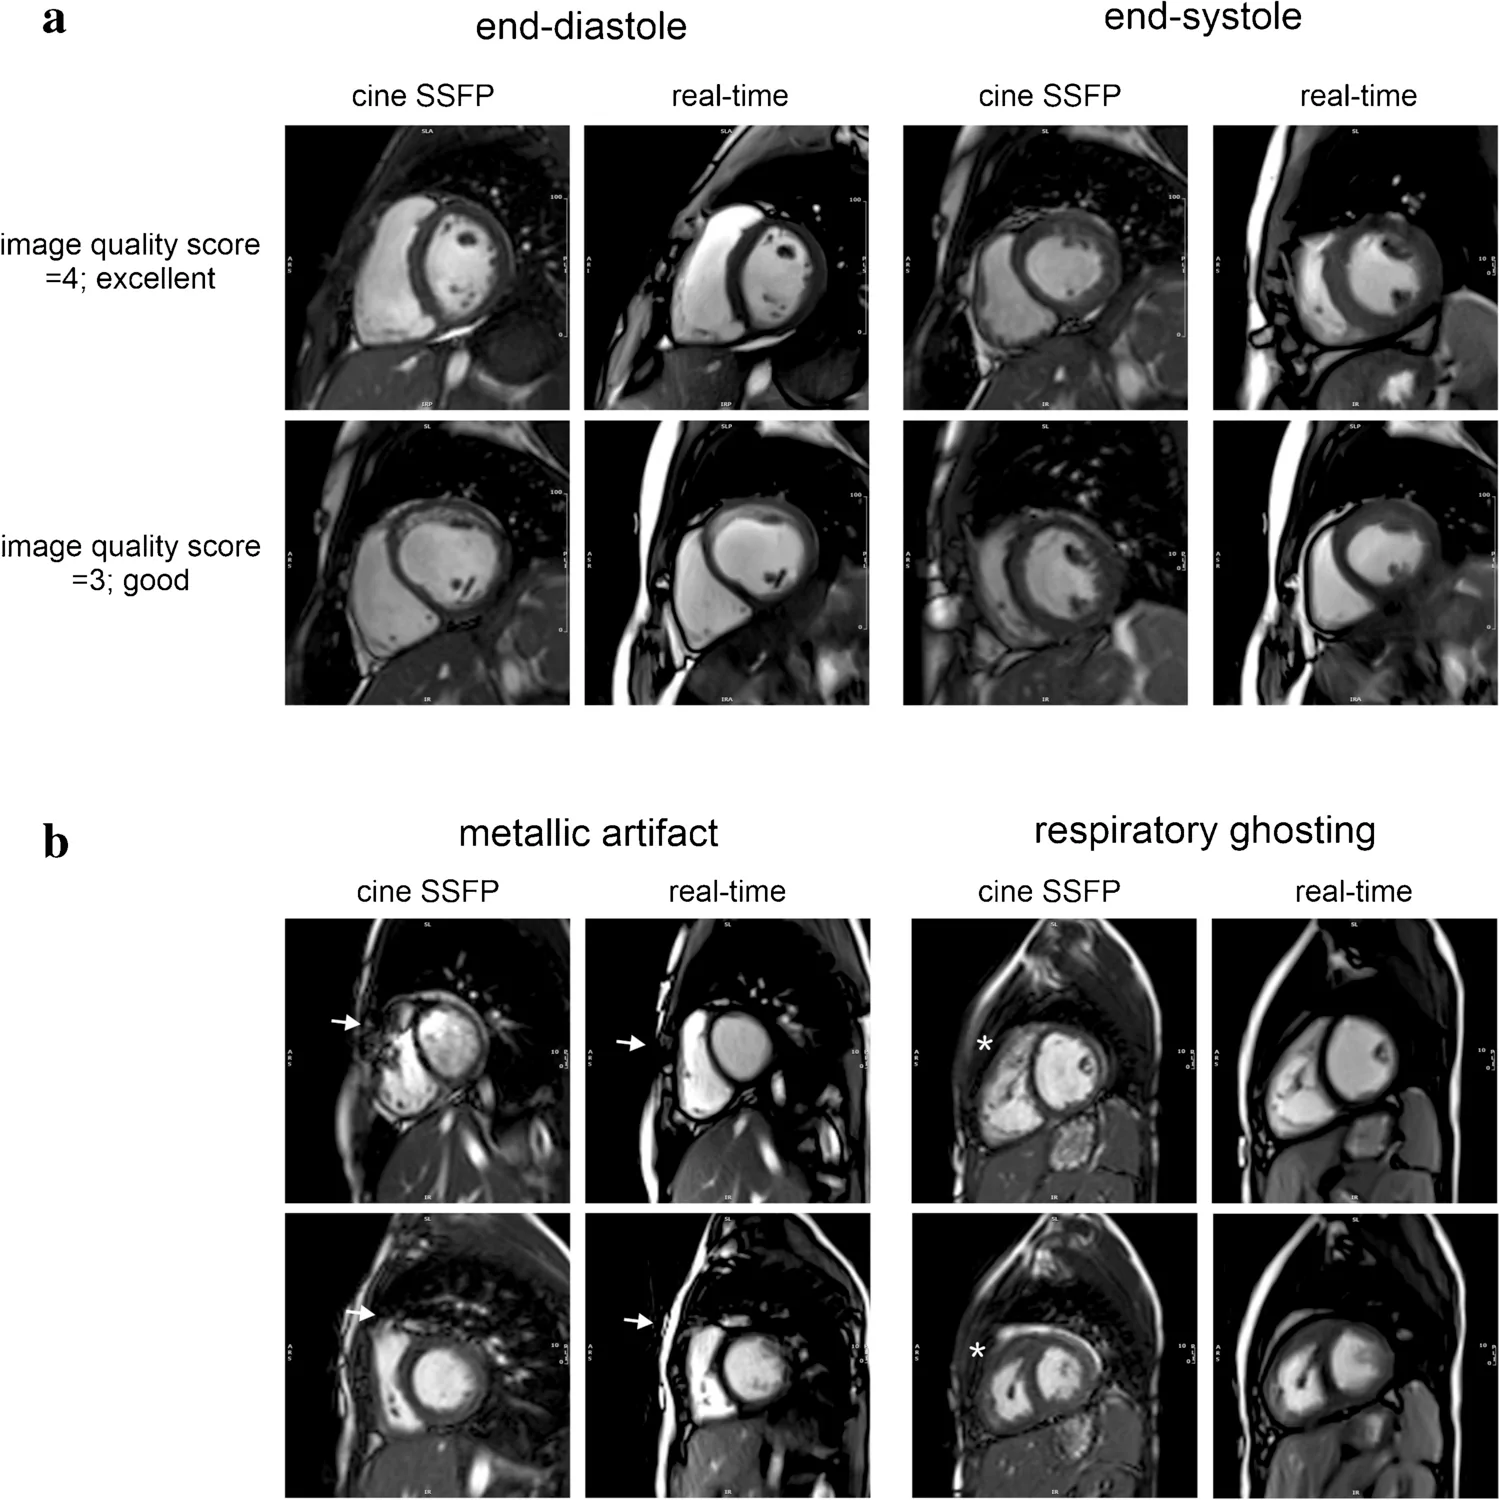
Image quality analysis. a, b Real time and conventional MR images
